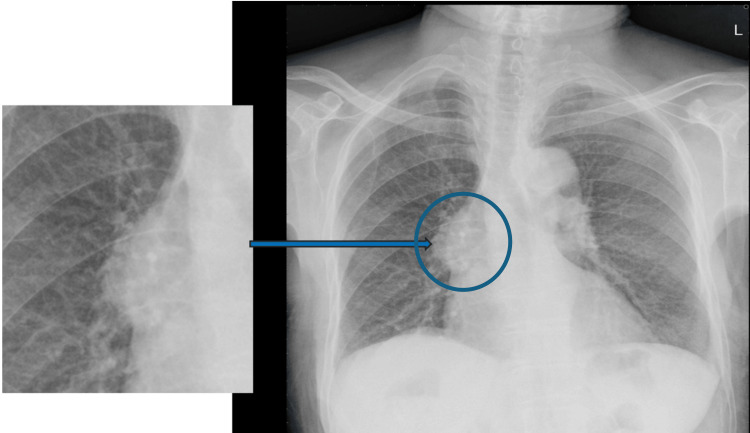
Postero-anterior chest x-ray of a 73-year-old male patient with no history of cough or previous TB shows a right perihilar mass with overlay sign (blue arrow) indicative of lymphadenopathy. Image Credit: Field data from the 2013 National TB Prevalence Survey. Permission was obtained from Dr. Frank Adae Bonsu, the Principal Investigator and the original data custodian of the 2013 National TB Prevalence Survey, to use the image.
